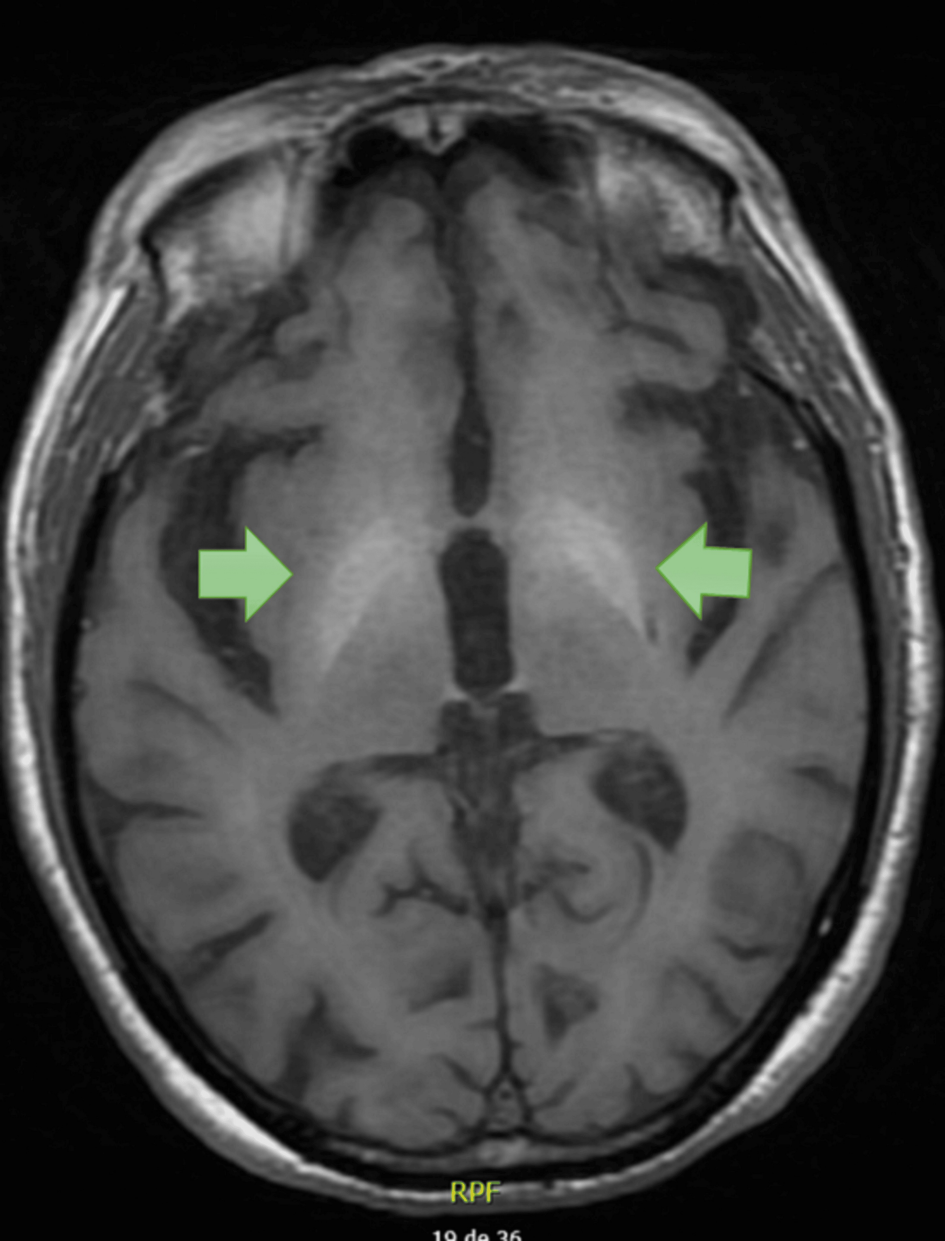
MRI of Patient 1 showing T1 hypersignal in the lenticular nuclei.
